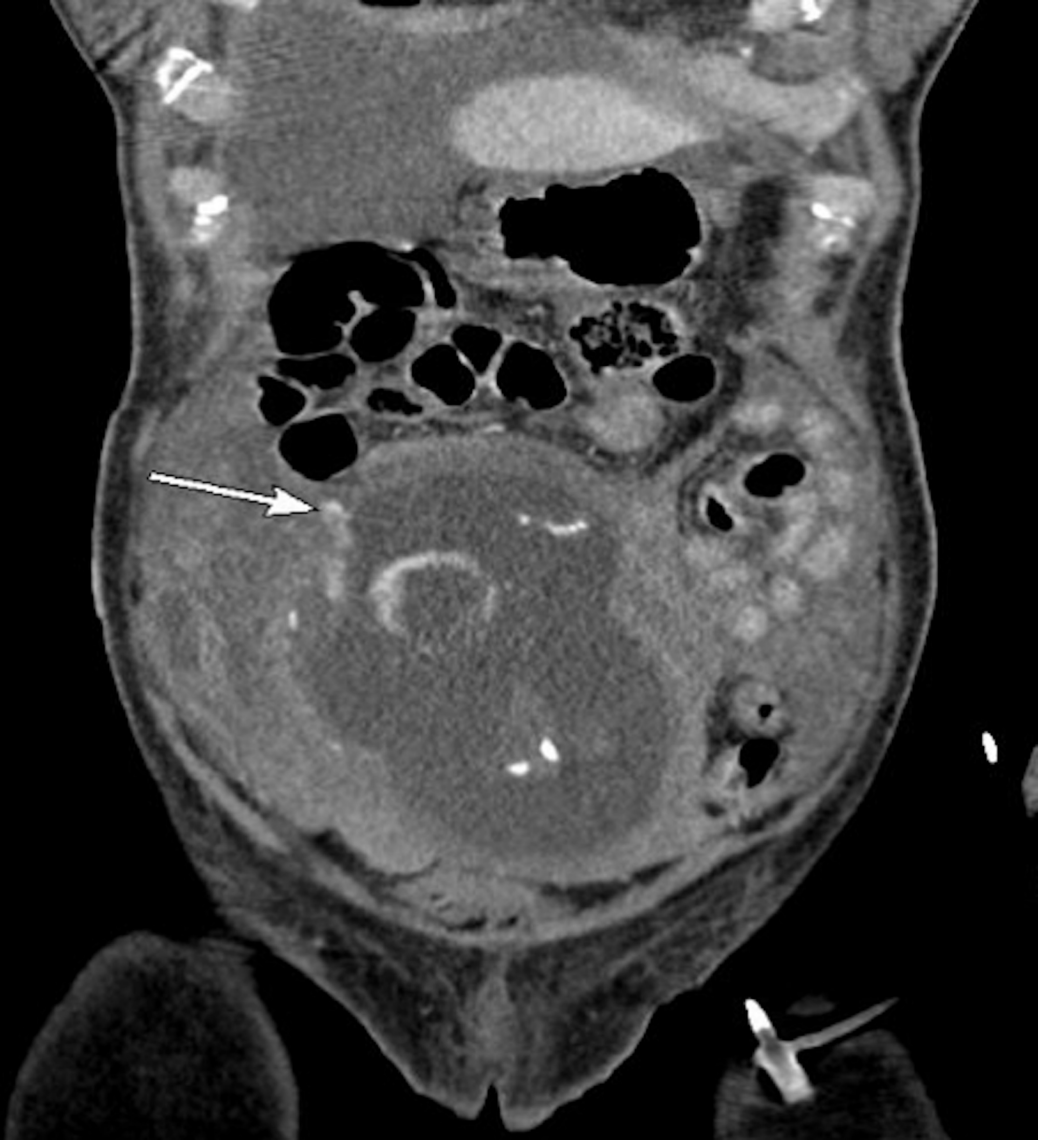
Computed tomography of the abdomen and pelvis. Coronal view showing active radiodense contrast extravasation from the perforated focal area of thinning of the right anterolateral fundus where there is a contrast blush (arrow).
